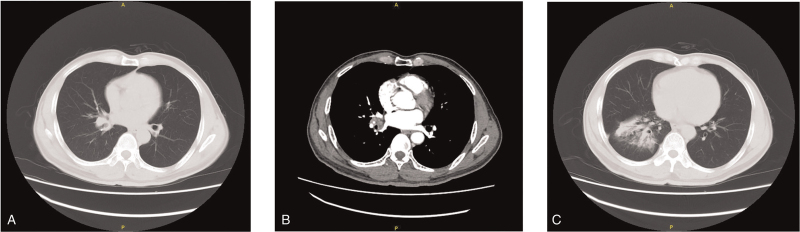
Computed tomography of the chest. A and B, computed tomography scan showing the bronchial lumen of the basal segment of the lower lobe of the right lung, which is narrow; soft tissue density nodules are seen around the same, with a range of approximately 2.4 × 1.3 cm. C, Multiple solid shadows are observed in the distal lung field.
